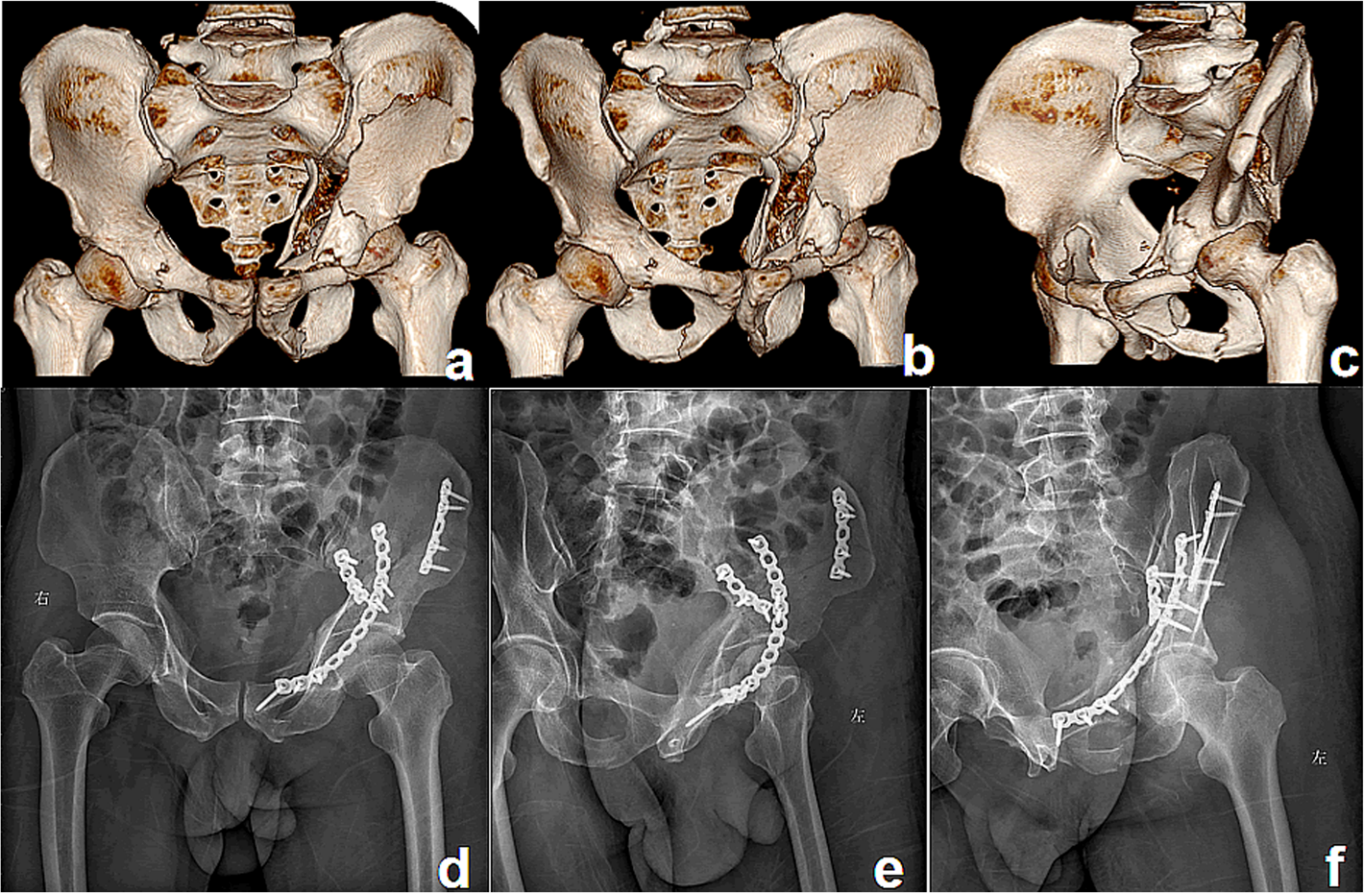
A patient treated with the IL approach. Preoperative anteroposterior (a), iliac oblique (b), and obturator oblique (c) 3D computed tomography reconstructions of a both-column fracture showing the severity of fracture displacement. Postoperative radiographs (d–f) showing good reduction and fixation
